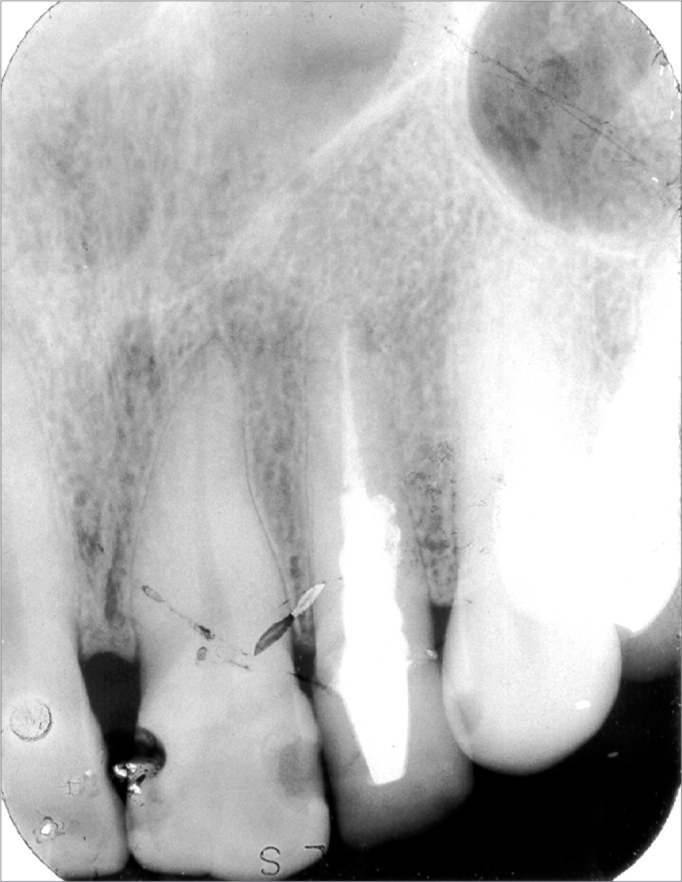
B-) Periapical radiograph of the central and upper left lateral incisors. This image shows no bone or dental alterations, which suggests a soft tissue lesion.
